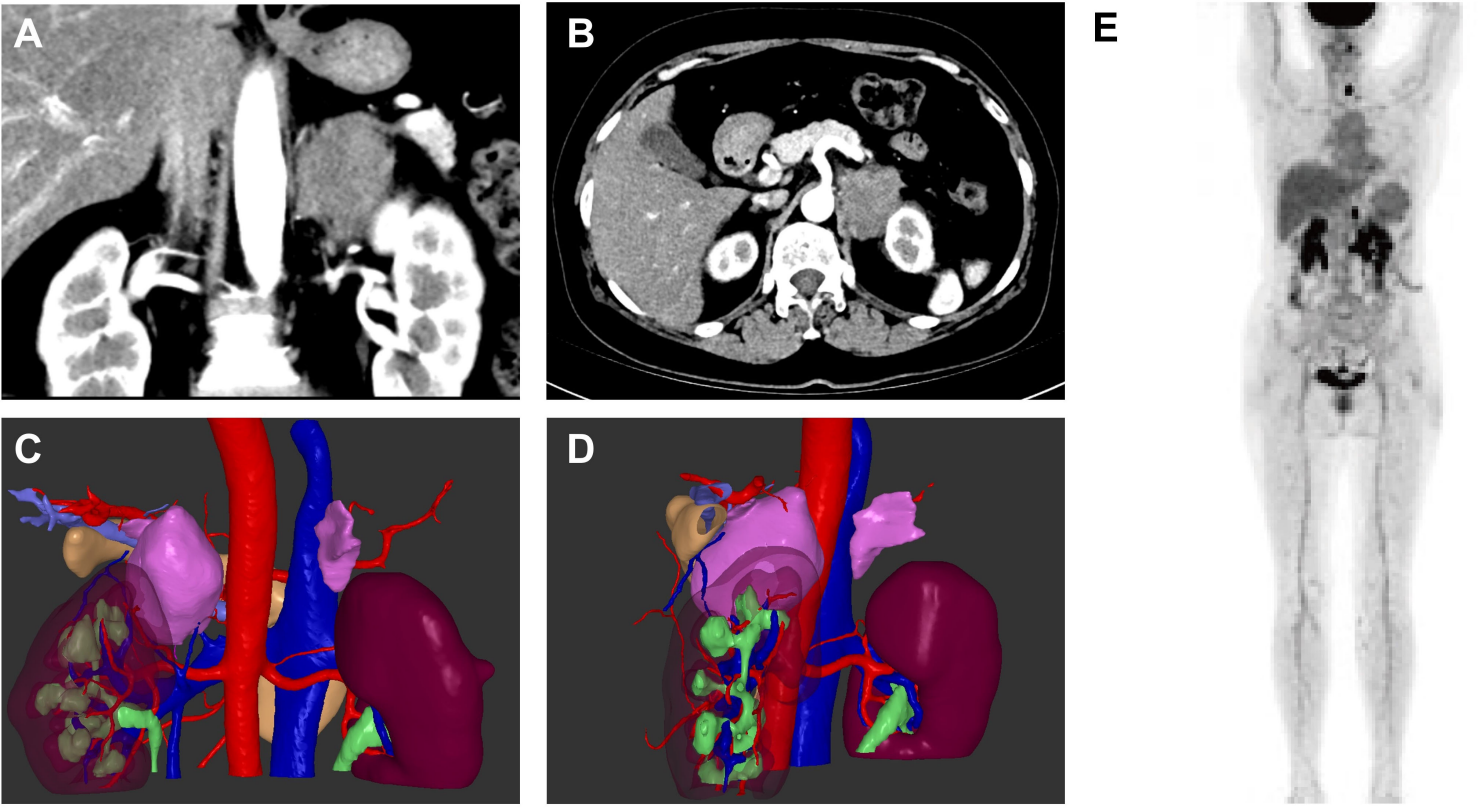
Enhanced computed tomography images of the left adrenal mass in sagittal and coronal views (A, B) and three-dimensional construction images (C, D). ^18^F-FDG PET/CT of the patient (E).
